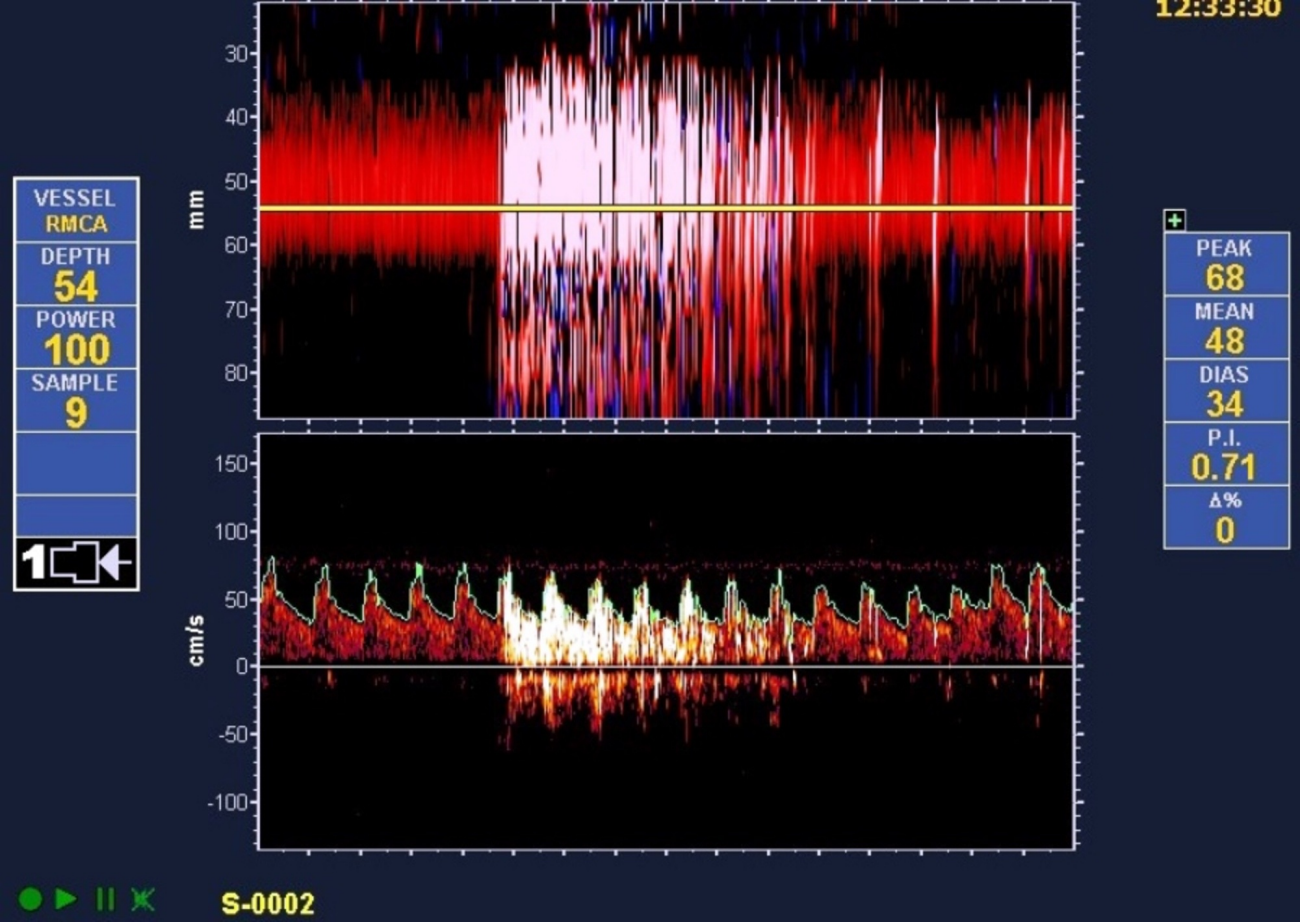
After an injection of agitated saline: transcranial Doppler of the right middle cerebral artery in a patient with a complex migraine with aura
